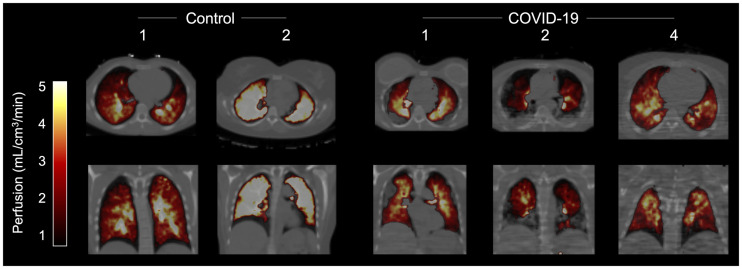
^15^O-water perfusion PET/CT images of 3 patients and 2 controls.
